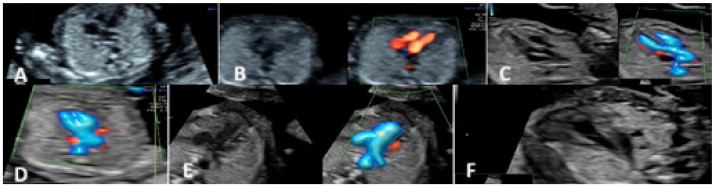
Second-trimester heart protocol. (A) Four-chamber view—Grey Scale. (B) Atrio-ventricular flows—Left side—Grey Scale and Right side—Color Doppler Mode. (C) Aorta—Left side in Grey Scale and Right side in Color Doppler Mode. (D) Crossing plane. (E) Pulmonary artery—Left side in Grey Scale and Right side in Color Doppler Mode. (F) 3-vessel and trachea view—Grey Scale.
